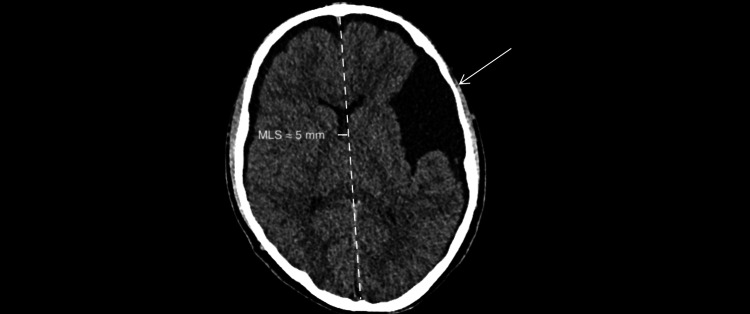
Non-contrast head CT (axial, brain window; W80/L40; 5-mm slices). Approximately 5-mm rightward midline shift, measured perpendicularly from the ideal midline (dashed line) to the displaced septum pellucidum, as indicated by the short perpendicular marker and label. Left middle-fossa arachnoid cyst (arrow).
